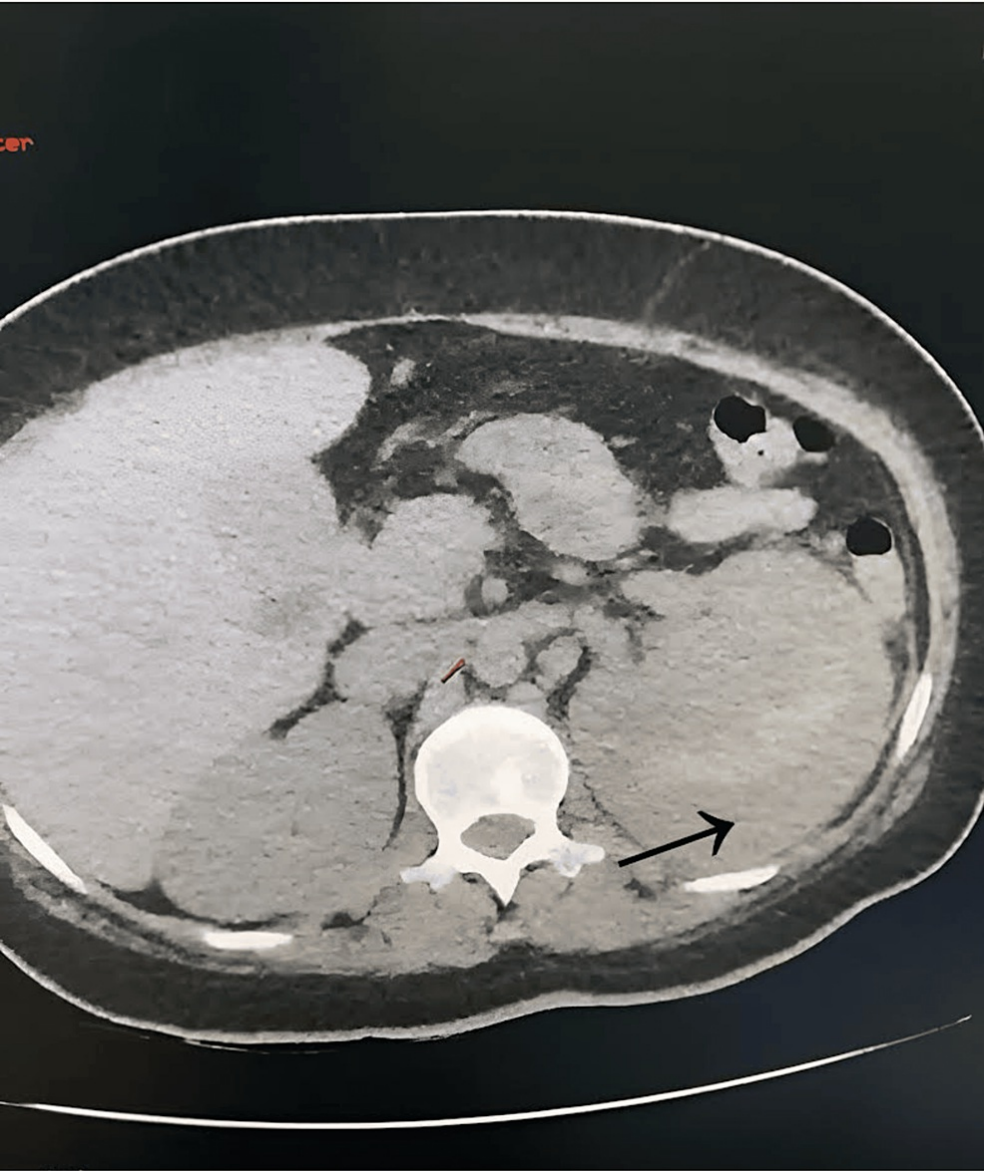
CT scan reveals the presence of a perirenal hematoma (black arrow) surrounding the kidney
